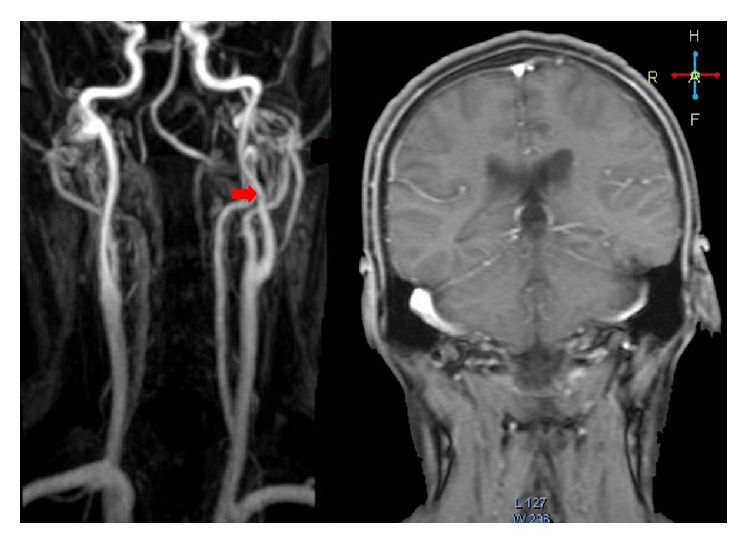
MRA and MRI with coronal slices demonstrating moderate narrowing of the left internal carotid artery just below the skull base (red arrow).
